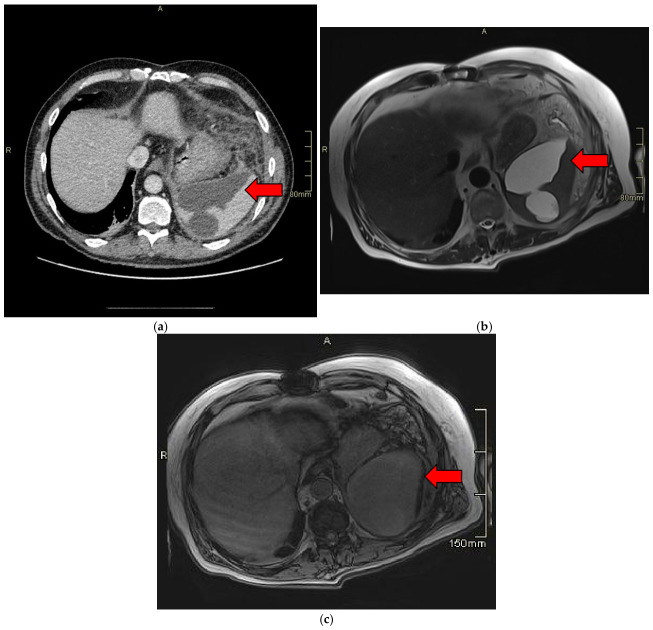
Pseudocyst in a 65-year-old male as a complication of acute pancreatitis (a) Axial contrast-enhanced CT of a well-circumscribed and unilocular hypodense pseudocyst with no overt calcifications or solid components. (b,c) Axial MRI T2-weighted image demonstrates a well-circumscribed, thin-walled, hyperintense splenic pseudocyst with no internal solid components.
